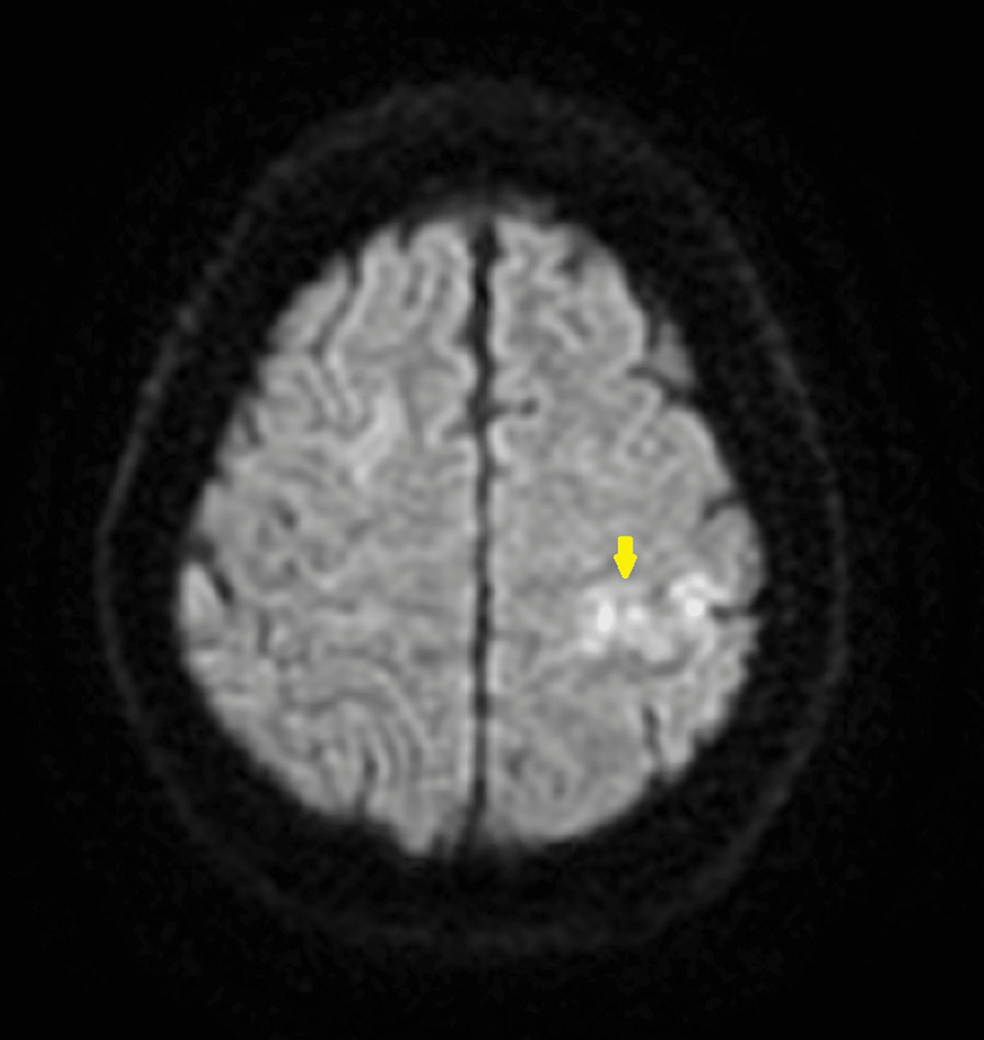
Axial view of MRI DWI sequence showing diffusion restriction signifying infarction MRI, magnetic resonance imaging; DWI, diffusion weighted imaging
